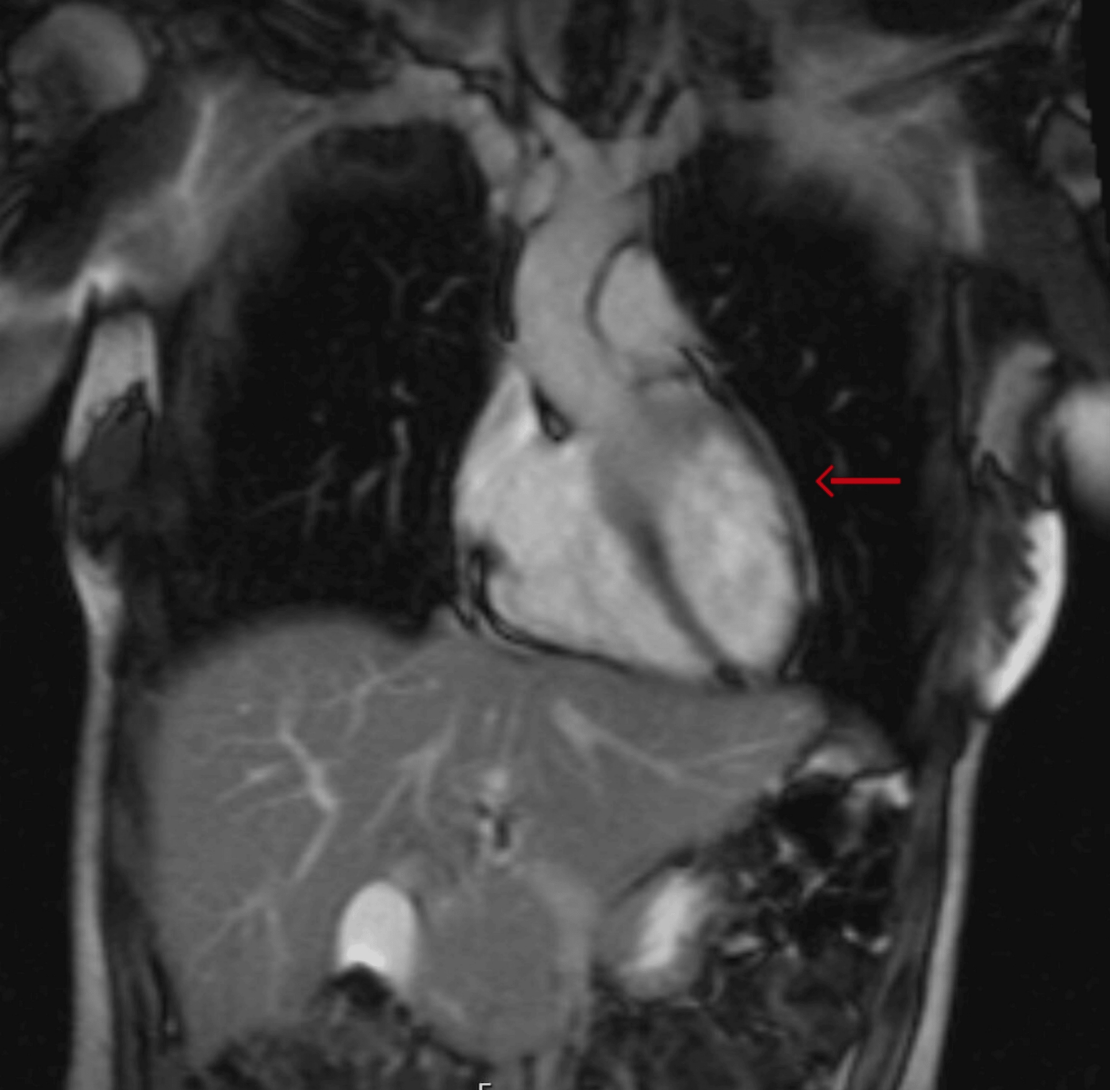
Cardiac MRI after two years, revealing normal pericardium (red arrow)
